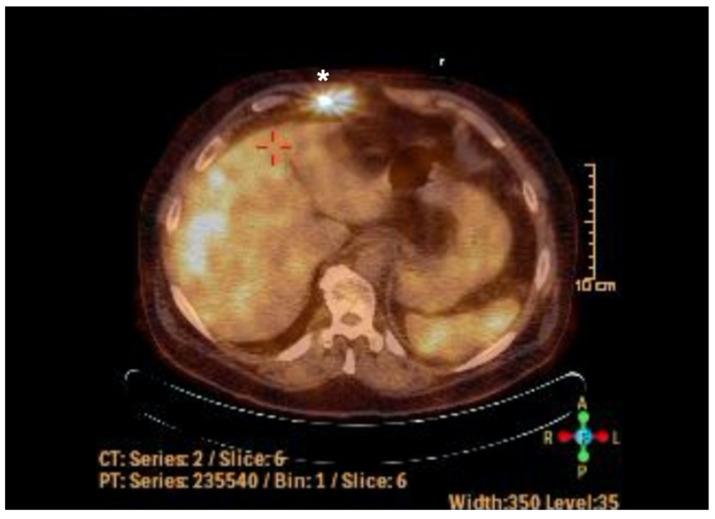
PET-CT scan of the upper abdomen before complex wound treatment. * PET positive driveline of the LVAD-System.
